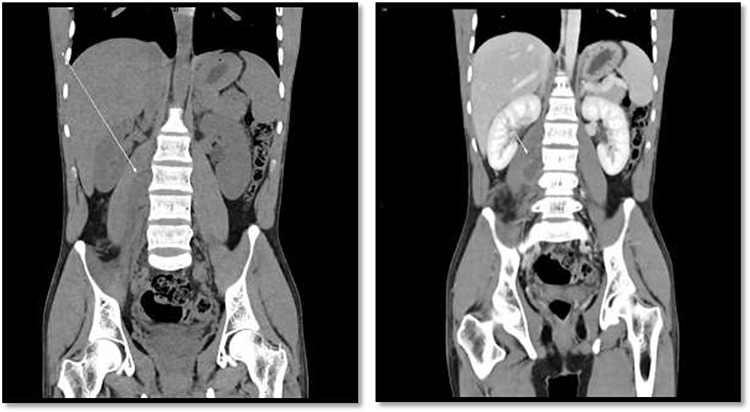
CT scan showed there is 4 × 3 cm lobulated mass noted at the right paravertebral space invading the right psoas muscle at the level of L3 and L4 and extending through the neural foramina, the associated with epidural component compressing the spinal cord from the level of the L2–L4.
